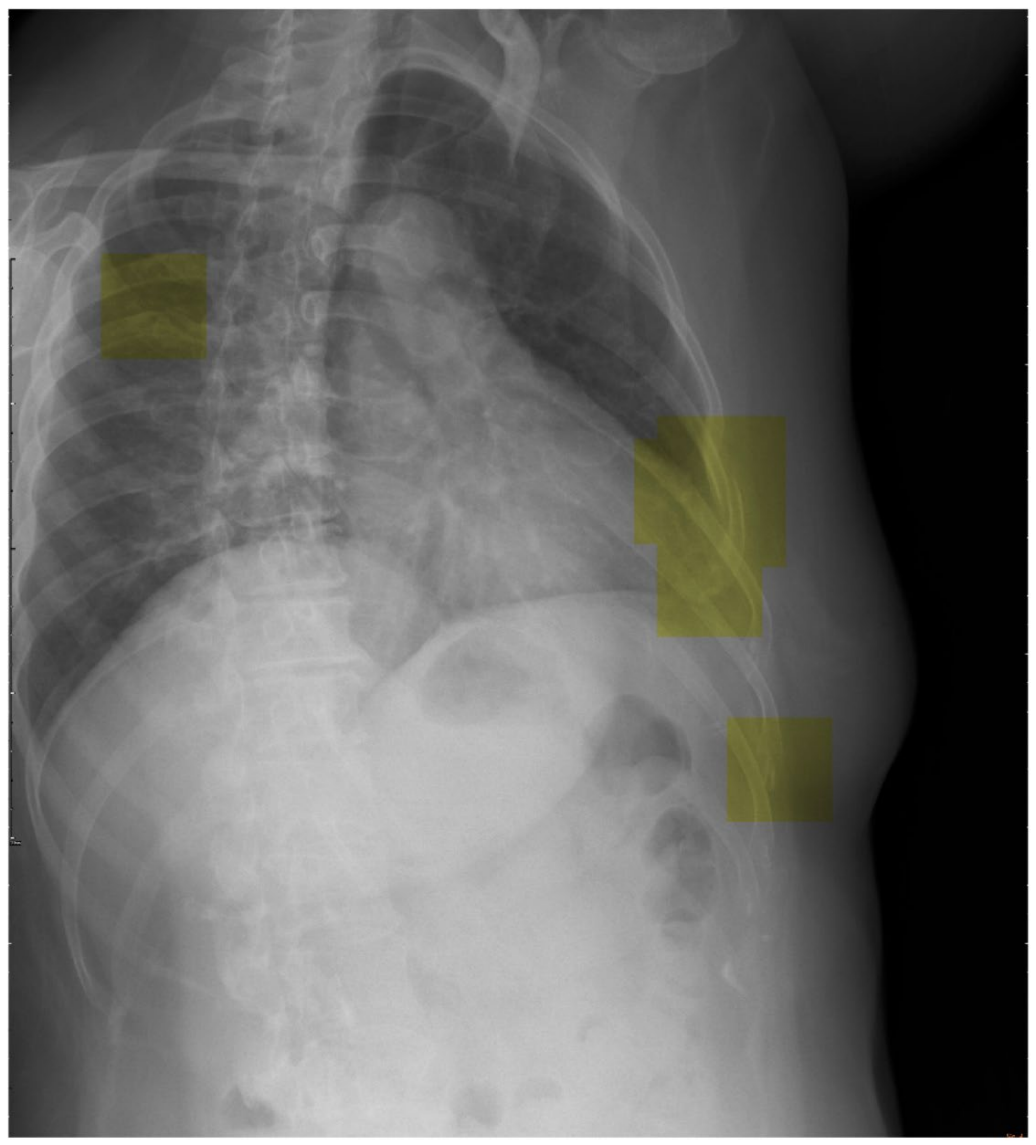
Visual representation showcasing the application of inference (highlighted by the yellow box) in our model for detecting potential rib fractures on chest radiographs.
